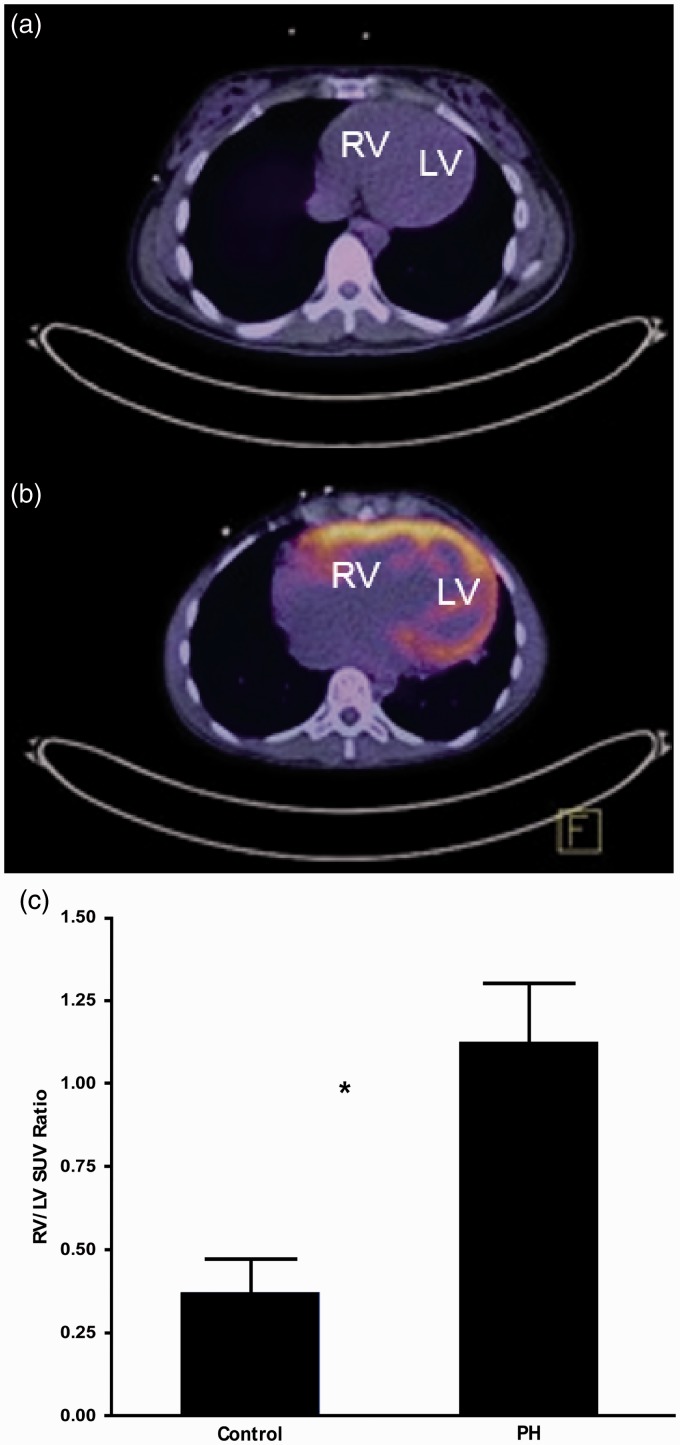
Fused PET/CT trans-axial slices of healthy control (a) and PH (B) participants. The control has minimal FDG uptake in the myocardium, whereas the PH participant has significant uptake, especially in the right ventricle. RV/LV SUV ratio in healthy controls and individuals with PH (c). Data are represented as mean ± standard error of the mean. Asterisk indicates statistically significant difference (*P* = 0.03). RV = right ventricle, LV = left ventricle, SUV = standardized uptake value.
